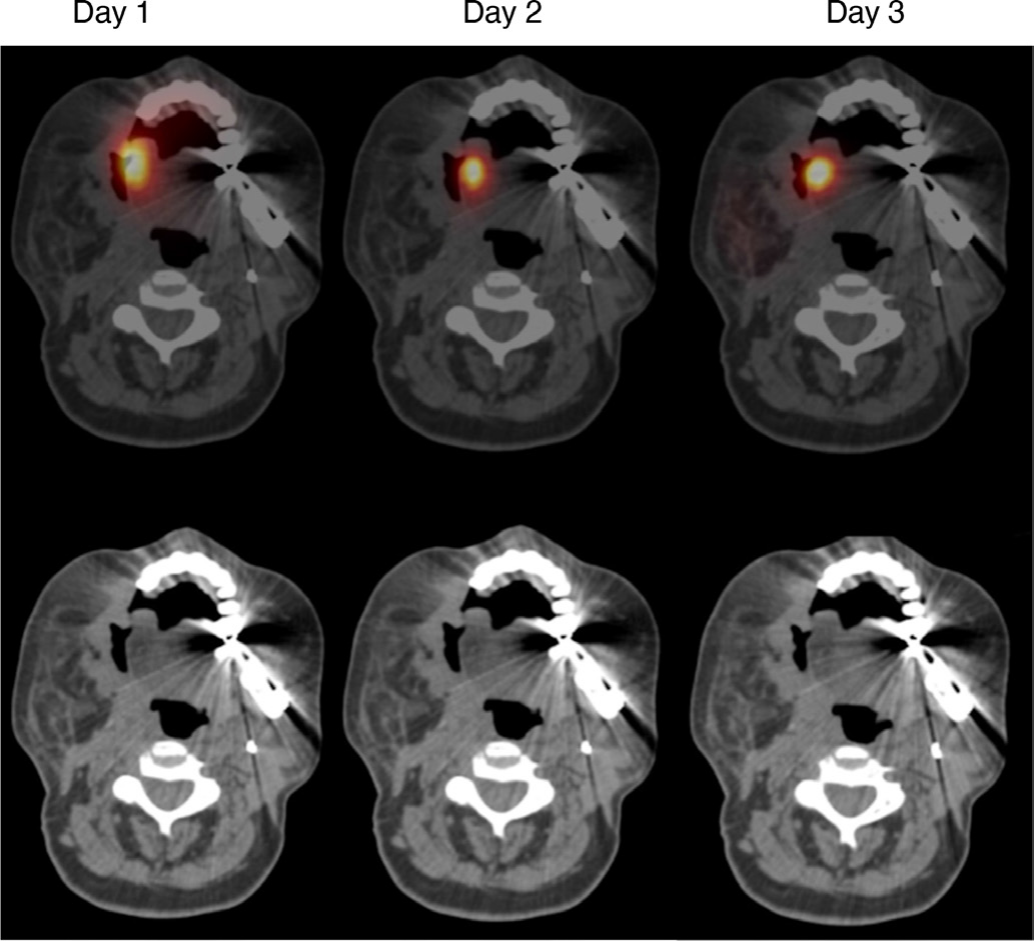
SPECT-CT imaging of [^111^In] radiolabeled T4 CAR T-cells. A radiotracer was prepared in which 30×10^6^ autologous T4^+^ CAR cells were labeled with 5MBq [^111^In] indium. The tracer was injected into a target lesion in the right buccal mucosa in a single subject (CAR-HNC-016). SPECT scans were performed at the indicated intervals and were coregistered with a CT scan (shown below) performed on day 1. CAR T-cells, chimeric antigen receptor T-cells; SPECT-CT, single photon emission-CT.
